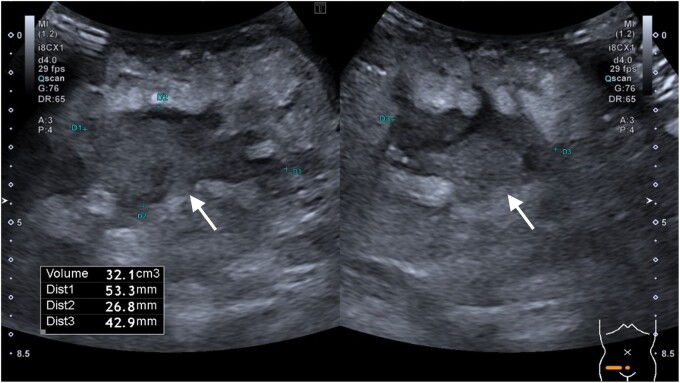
Transverse and longitudinal ultrasound images of the right groin showing an ill-defined fluid collection with surrounding echogenic fat (arrows).
